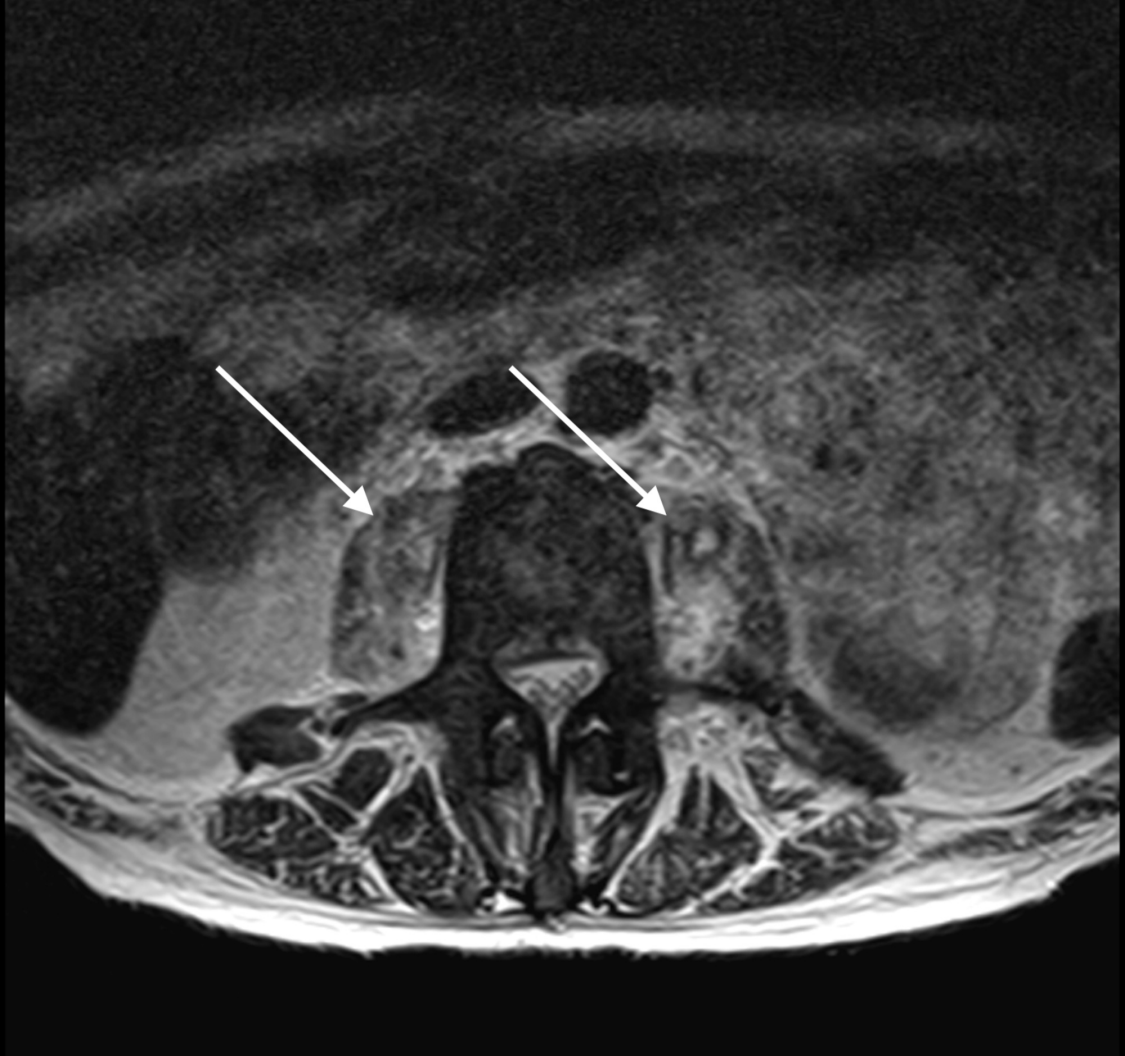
Magnetic Resonance Imaging Without Contrast of the Lumbar Spine Axial image of the lumbar spine revealing decreased size of the abscesses (see arrows) in the psoas muscles bilaterally
